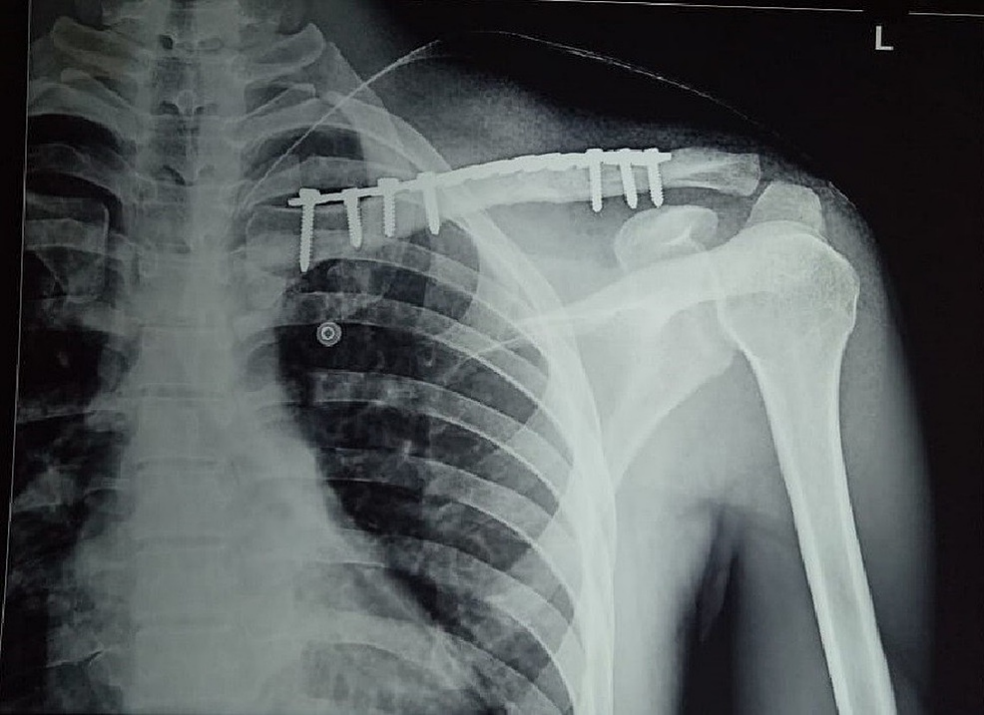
Radiograph of the left clavicle showing united fracture with locking plate in situ
